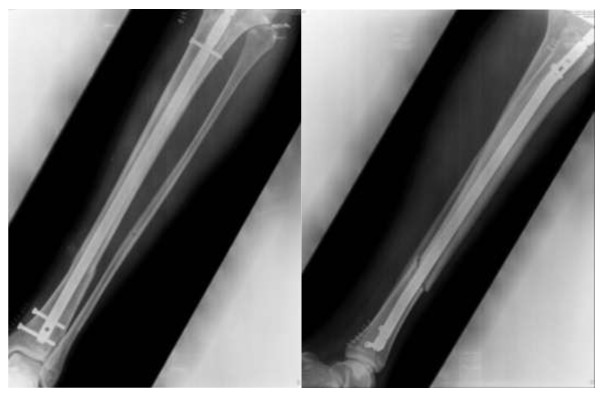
Anteroposterior and lateral radiographs of the left tibia after nailing.
